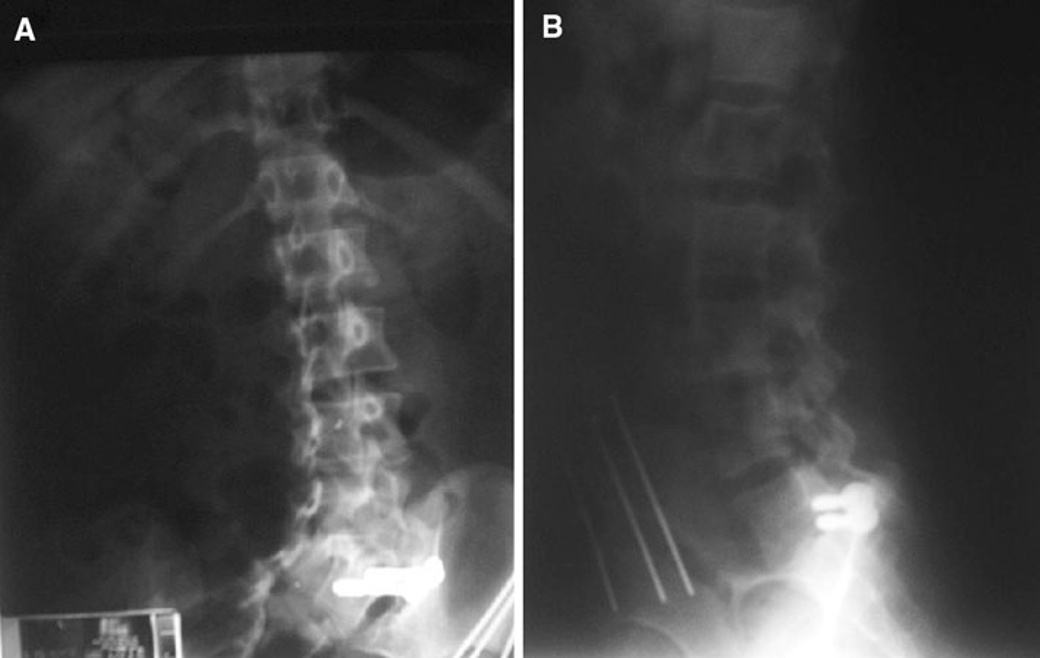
a and b. Posterior lumbo-pelvic fusion by percutaneous CT-guided application of screws
